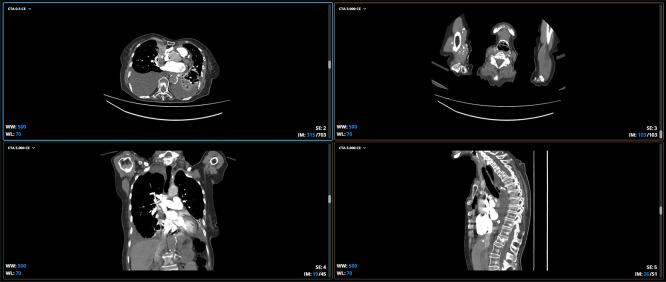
Sample of the dataset with a multislice computed tomography angiography (CTA) study with intravenous contrast covering the chest and neck region.
